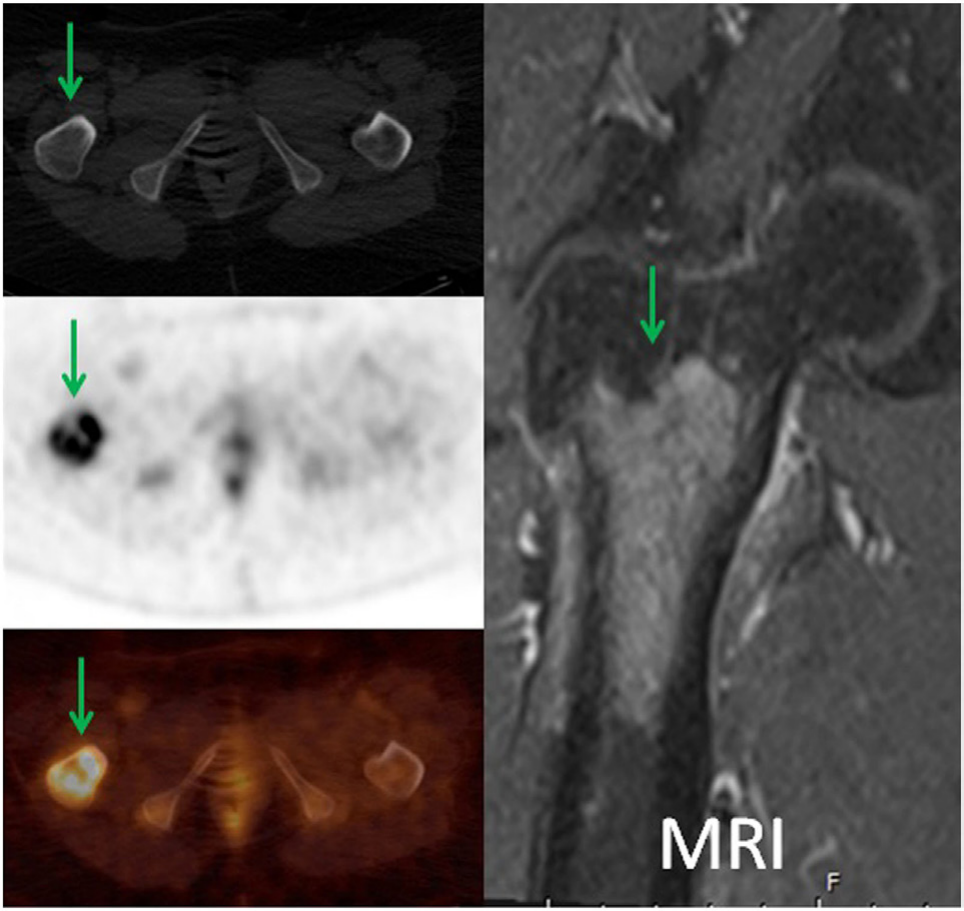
A 57-year-old female diagnosed with chronic myelogenous leukemia status post allogenic bone marrow stem cell transplant and widespread disease. 18F-FDG PET demonstrated intense focal uptake in the intertrochanteric region of the proximal right femur with no corresponding bone changes in the CT (green arrows). The patient had MRI with contrast which demonstrated marrow replacing lesion. Patient received XRT, BMA, and had orthopedic fixation. In addition, 18F-FDG PET directed the biopsy site for this patient.
